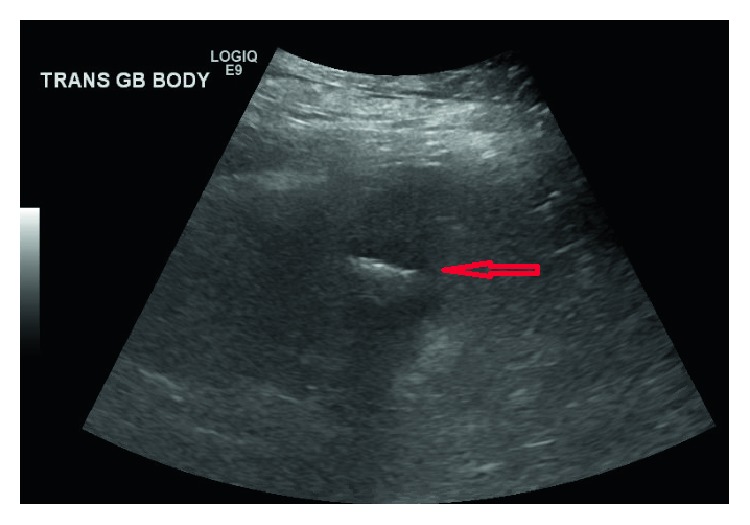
Abdominal ultrasound depicting a large stone in the gallbladder (red arrow).
